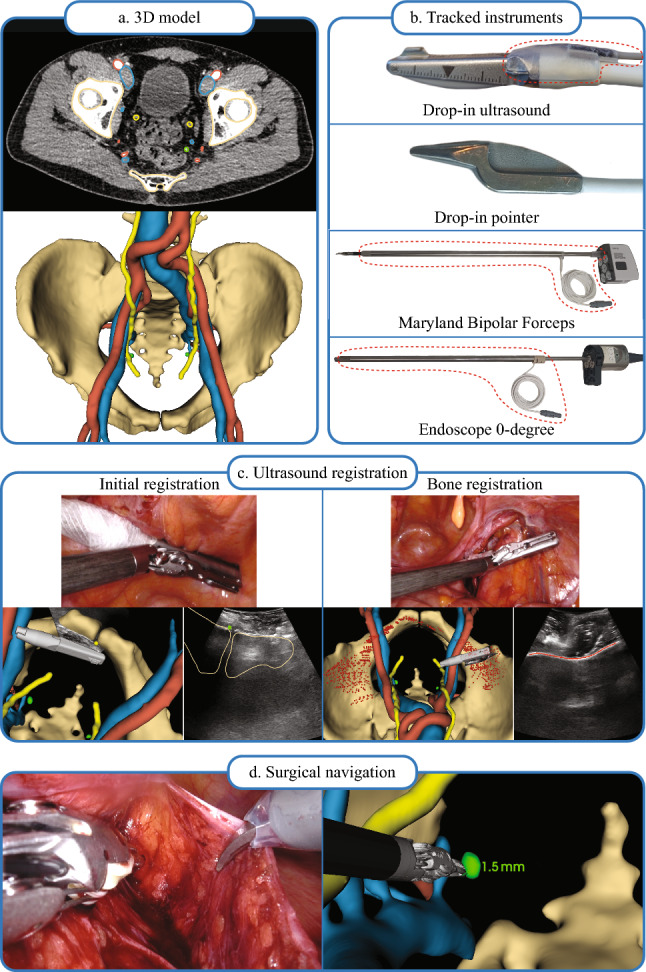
Overview of the navigation workflow. a 3D model delineated from a preoperative CT scan with two target lymph nodes in green. b Tracked instruments used during surgery (red dashed line highlights the tracker attached to the device). c Initial registration using a point selected on the pubic bone in CT (yellow point) and ultrasound (green point) & bone registration using automatic segmentation of the bone surface on ultrasound (red line and points). d Navigation view showing the distance of the tracked instrument to the target lymph node (green)
